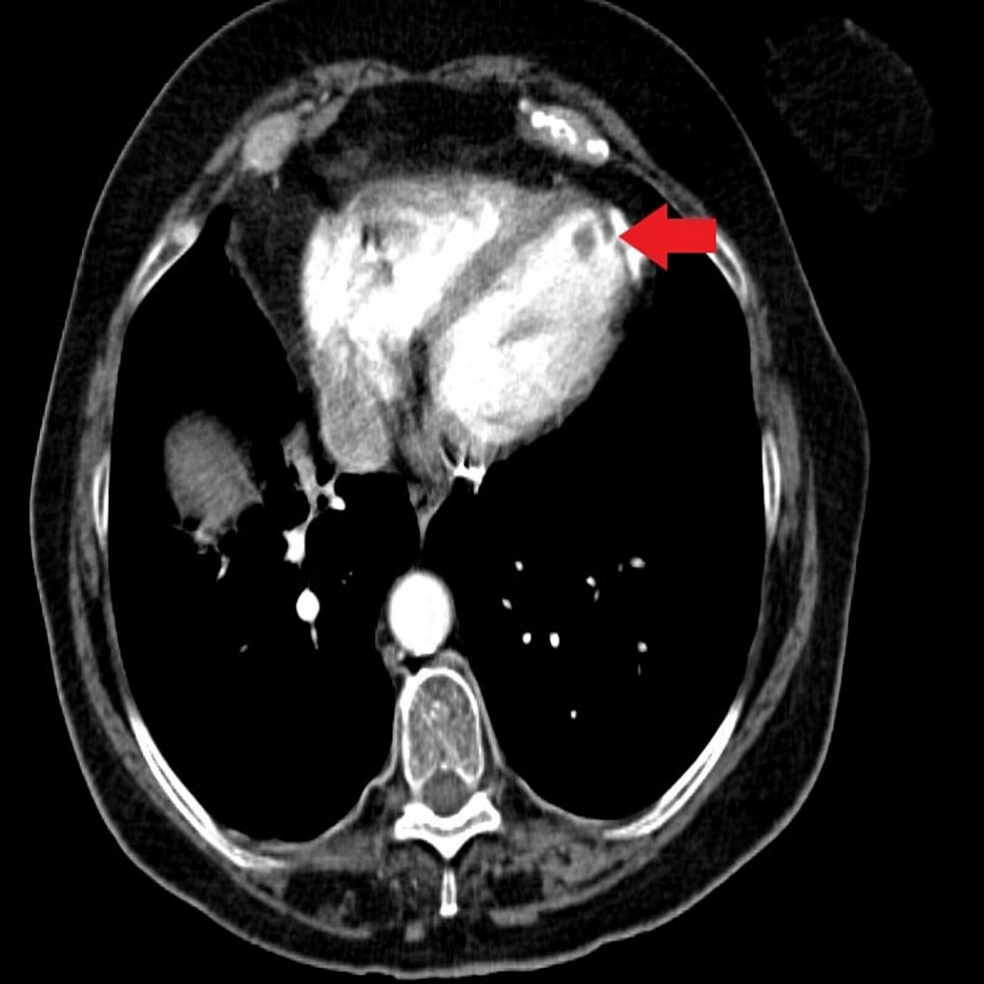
CT chest showing clot at the left ventricle
